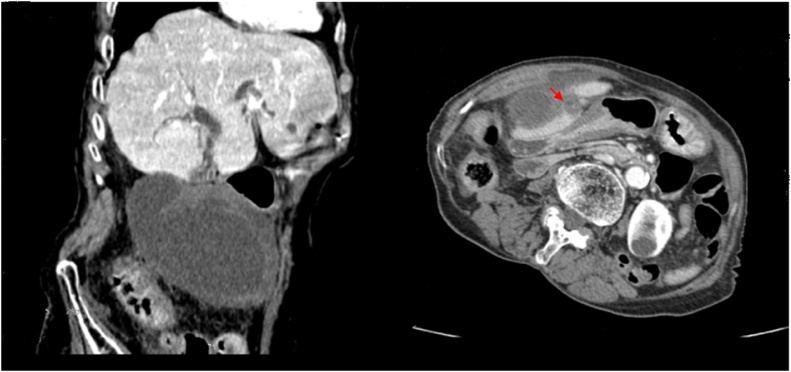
Abdominal CT revealed a distended, floating gallbladder located outside its normal fossa, with thickened wall and twisted pedicule (red arrow).
